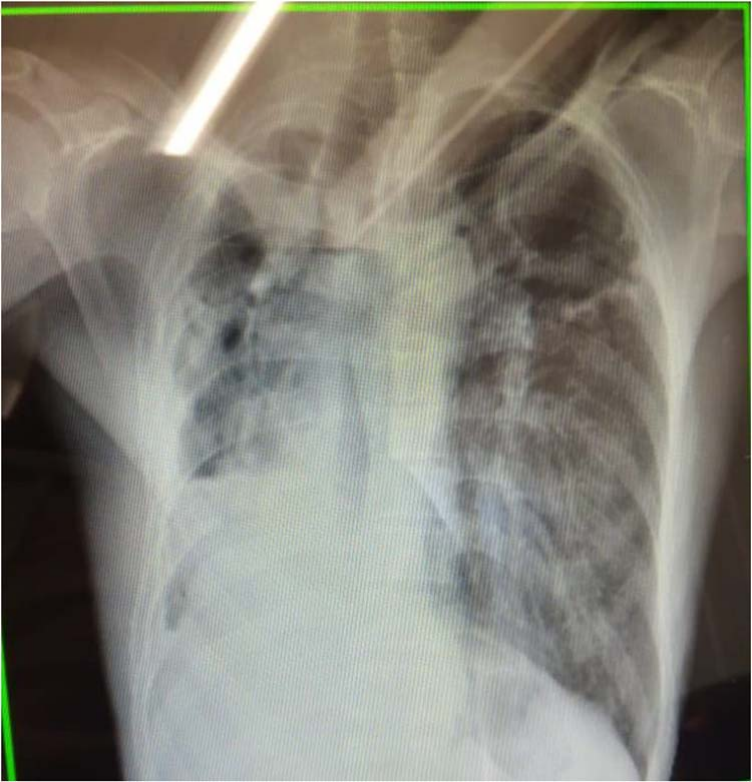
A chest X-ray which was taken before starting treatment with cavities more prominent to the left than right lung.
